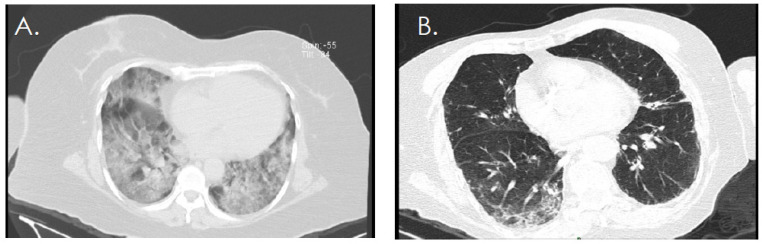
Representative chest-computed axial tomography (CT) slices. Each patient was screened based on anatomical abnormalities observed on chest CT scans. (A) Chest CT findings categorized as CO-RADS 5 and (B) chest CT findings classified as CO-RADS 3. CO-RADS, COVID-19 Reporting and Data System.
